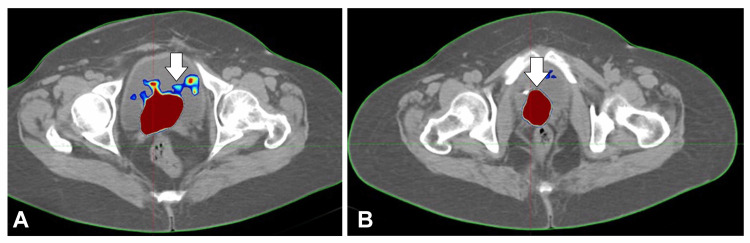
(A,B) Images of the fused PET scan with MRI showing the recurrent disease in the vault of the patient (white arrows) PET: positron emission tomography; MRI: magnetic resonance imaging
